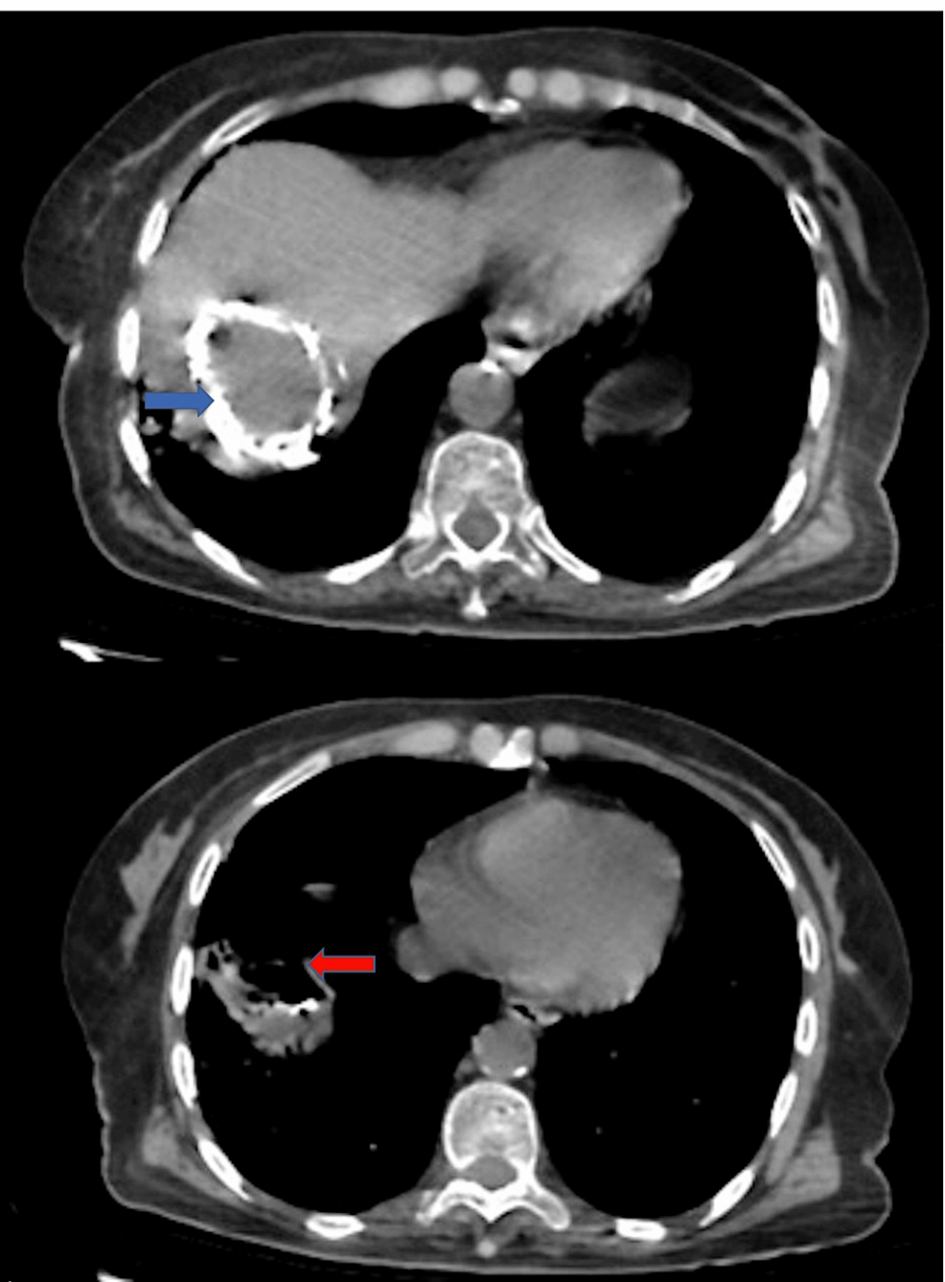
Thoraco-abdominal CT scan with and without contrast showing a bilio-bronchial fistula (red arrow) associated with a hepatic cystic lesion of segment VII (blue arrow).
